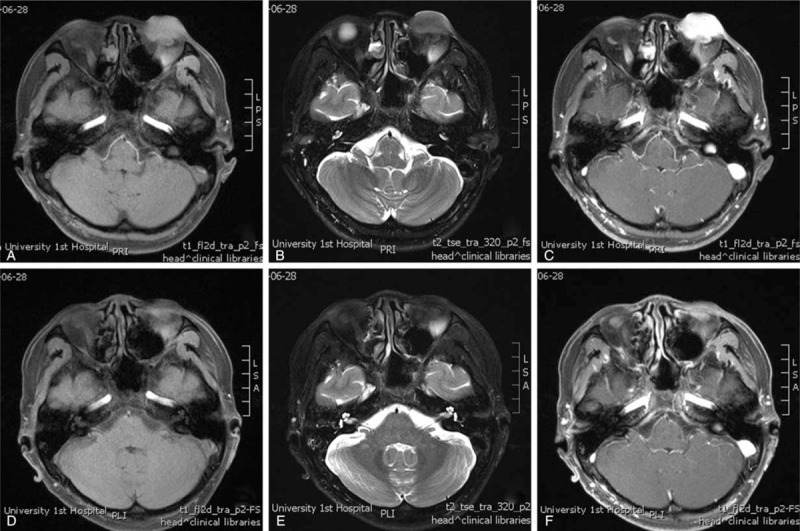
Magnetic resonance imaging (MRI) of the eye. A, B and C: The MRI of the eye before radiotherapy showed an abnormal signal of lumps in the lower left eyelid on T1 and T2 imaging; D, E and F: The MRI of the eye at 5 months after radiotherapy showed a slightly abnormal signal in the lower left eyelid on T1 and T2 imaging.
